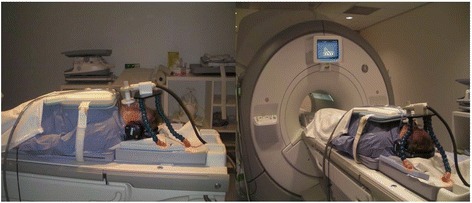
Line-up during the MRI Patients were placed in supine position in the MRI scanner with an MRI-compatible spirometer positioned just above the head.
